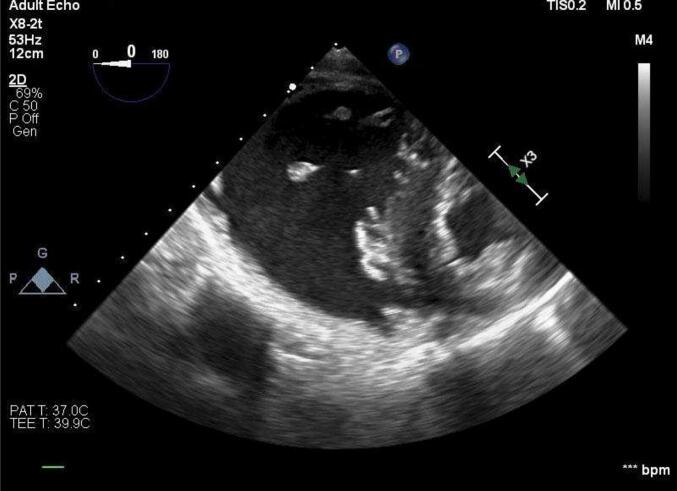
Transesophageal echocardiogram demonstrates dilated right ventricle indicating right heart strain.
